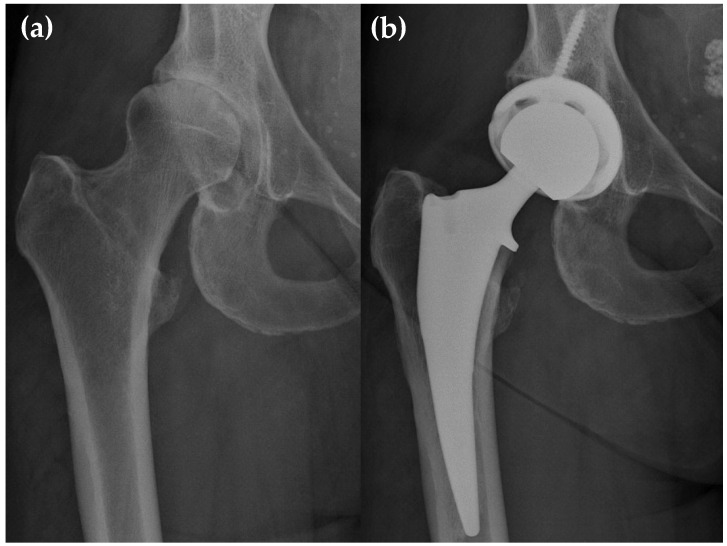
Representative case of primary THA using a collared, triple-tapered femoral stem: (a) Preoperative radiograph of the right hip showing end-stage primary osteoarthritis; (b) one-year postoperative radiograph demonstrating appropriate implant positioning and stable fixation without evidence of subsidence, loosening, or periprosthetic fracture.
